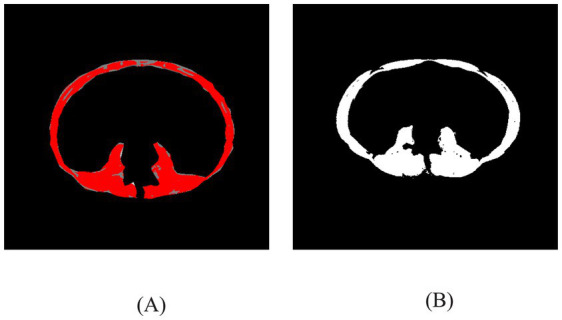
Semi-automatic segmentation of skeletal muscle area at the third lumbar vertebra (L3) level. (A) CT image of a patient with sarcopenia, showing reduced muscle mass (red area). (B) CT image of a patient without sarcopenia, showing preserved muscle mass (white area).
